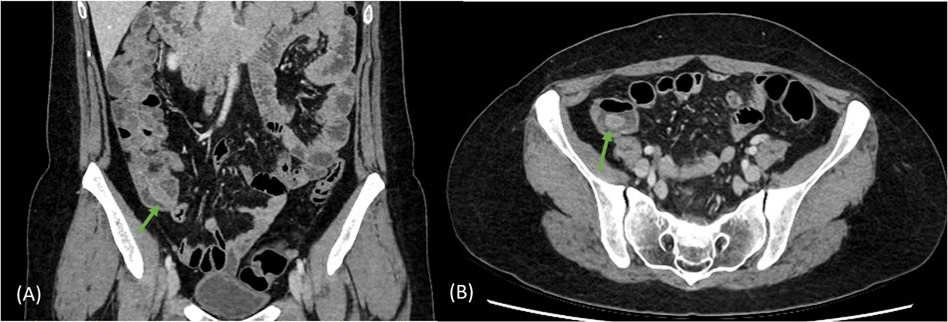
Coronal (A) and axial (B) views of CT scan demonstrating tubular rim enhancing structure in the caecal lumen with surrounding fluid, suspicious for invaginated appendix, which was otherwise not identified.
